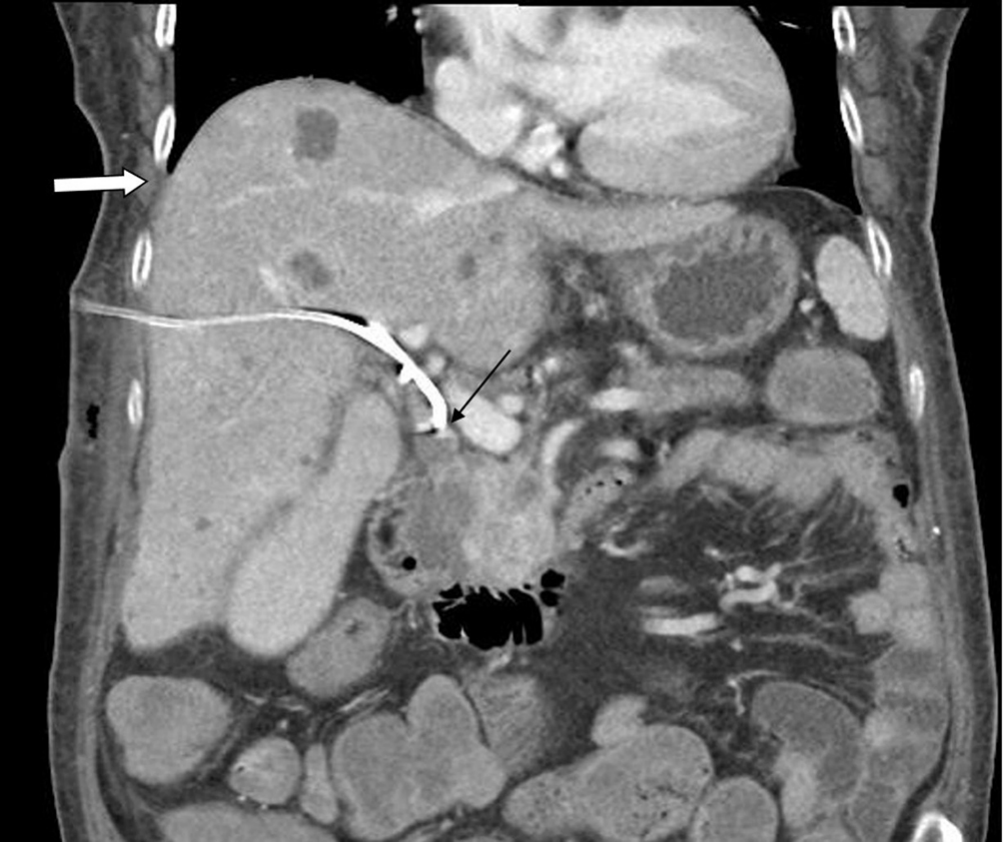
Coronal view of the post enhancing abdominal computed tomography reveals the tip of the catheter in the common bile duct (thin arrow), and without right pleural effusion (thick arrow).
